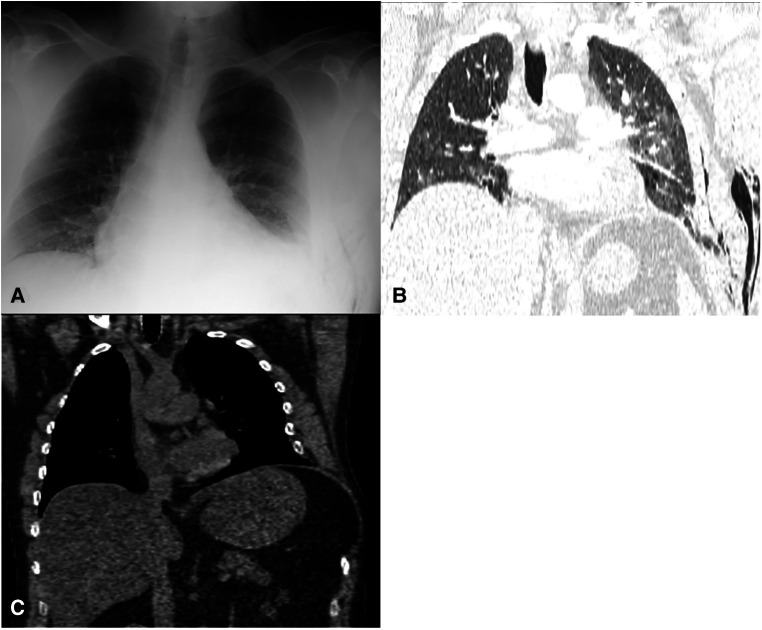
36-year-old man, BMI 40.3, with a left SLIH presenting with extensive bruising, abdominal pain, and shortness of breath. (A) chest x-ray at presentation showed an subcutaneous emphysema within the left axillary region and supraclavicular fossa. (B) CTPA revealed a moderate left pneumothorax without tension, a left abdominal wall and neck subcutaneous emphysema, left effusion, and a displaced fracture of the left lateral 8th rib. Lung herniation was not reported; however, on a retrospective review, a small left-sided lung hernia could be identified. (C) A CT scan revealed a herniation of a small lingula portion, abdominal fat, and lateral aspect of the diaphragm, and old rib fractures of the 6th lateral rib, 7th non-united lateral rib with a 15-mm gap, and 8th posterior rib with callus formation with displacement of the anterior portion of the eighth rib more anteriorly with a wide separation of the distracted fragments.
